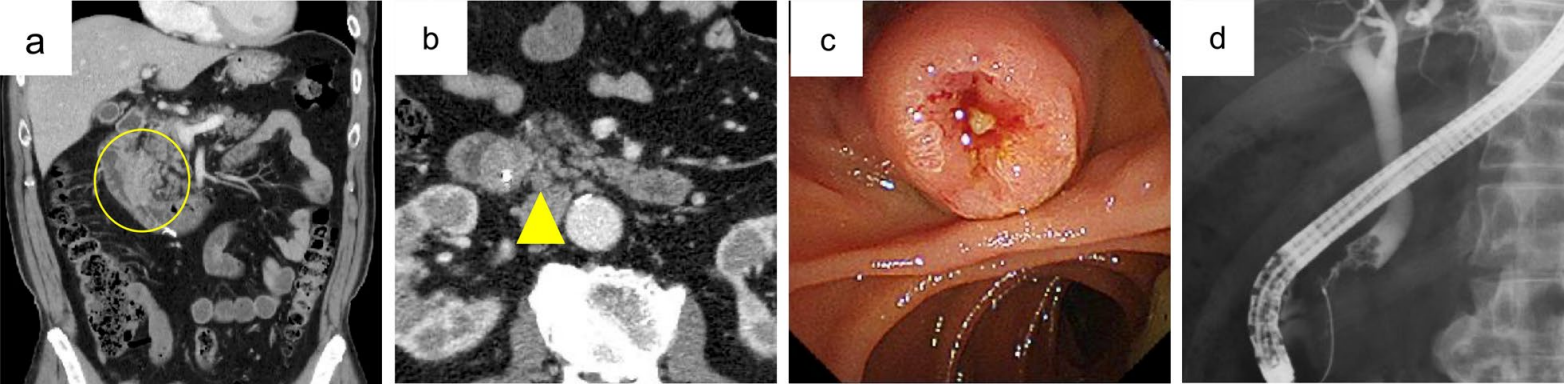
Preoperative examination. a, b Enhanced computed tomography image showing an enhancing tumor in the ampullary region protruding into the second part of the duodenum (35 mm × 51 mm) (circle) and an enlarged lymph node adjacent to the peripancreatic lesion (arrowhead). c Duodenoscopy image showing an ulcerated lesion in the swollen ampulla of Vater. d Endoscopic retrograde cholangiography image showing a mass in the distal bile duct arising from the papilla of Vater
